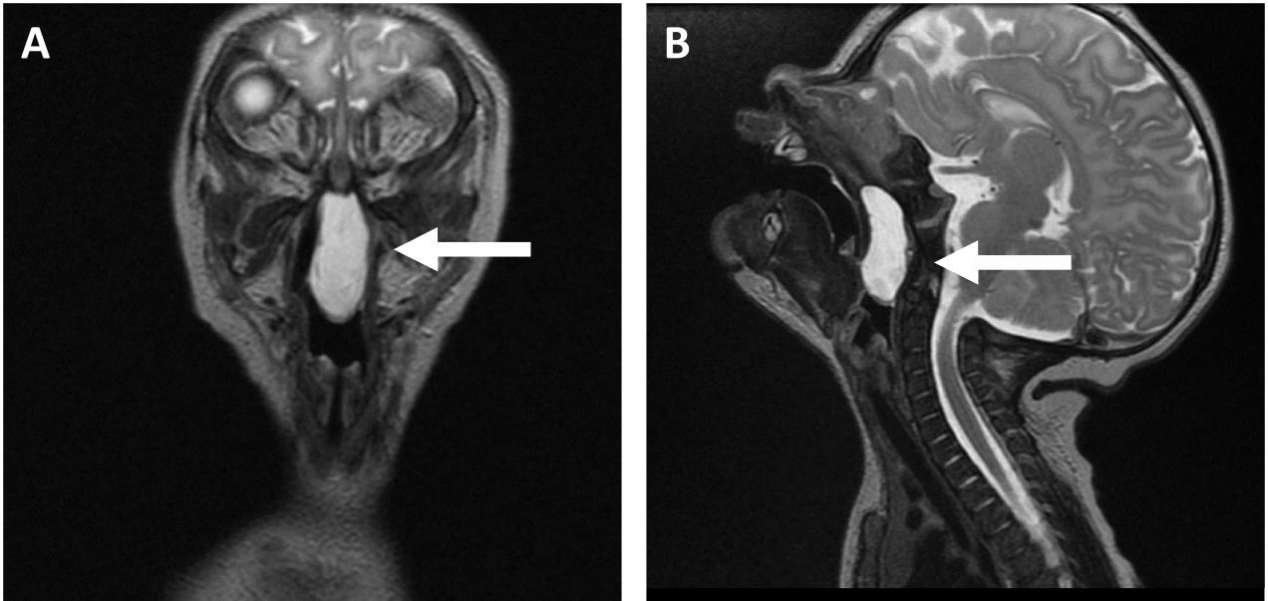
MRI with a Midline Nasopharyngeal Cystic-elliptical Mass, with Well-defined Borders and No Intra-cranial Involvement. A: Lesion begins in the posterior aspect of the nose and reaches the oropharynx. B: Sagittal plane.
